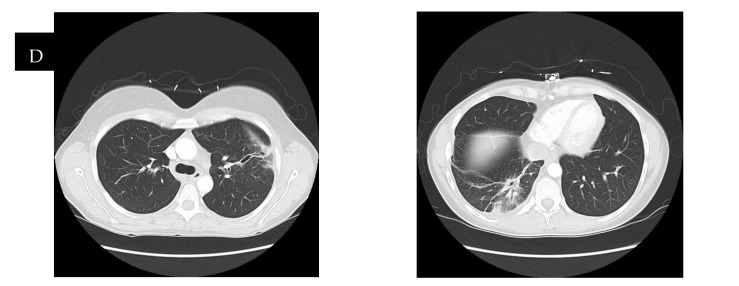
CT scans of a patient with necrotizing pneumonia and progression through time, with row (D) representing images after the completion of treatment. The time between images in row (A) and row (D) is six weeks. Row (B,C) represent CTs that were taken in between the time of row (A) and row (D), showing the progression of improvement.
